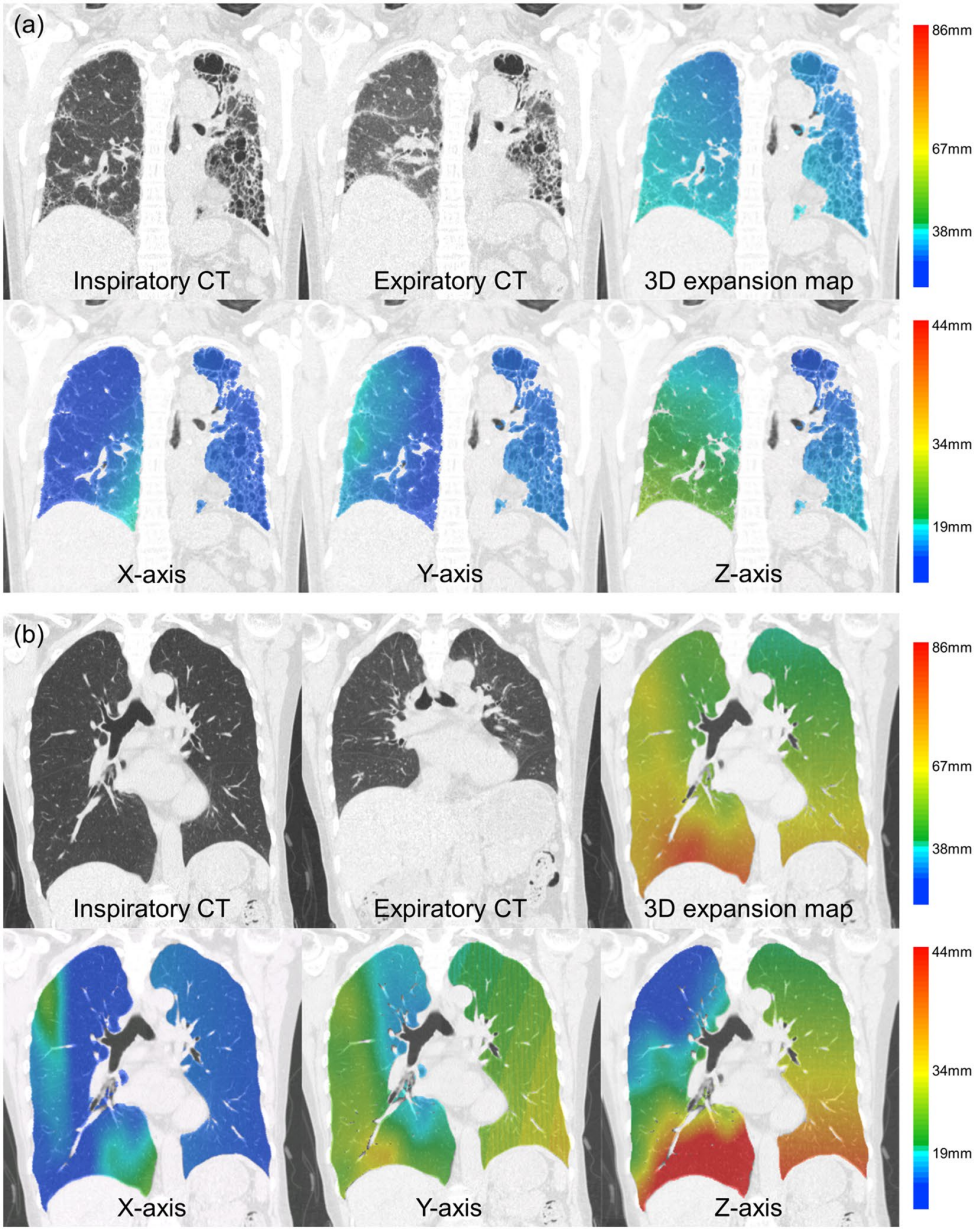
Representative images of paired full-inspiratory, full-expiratory CT images and lung expansion map in 3D and x-, y-, z-axis in (a) interstitial lung disease patient and (b) normal control.
